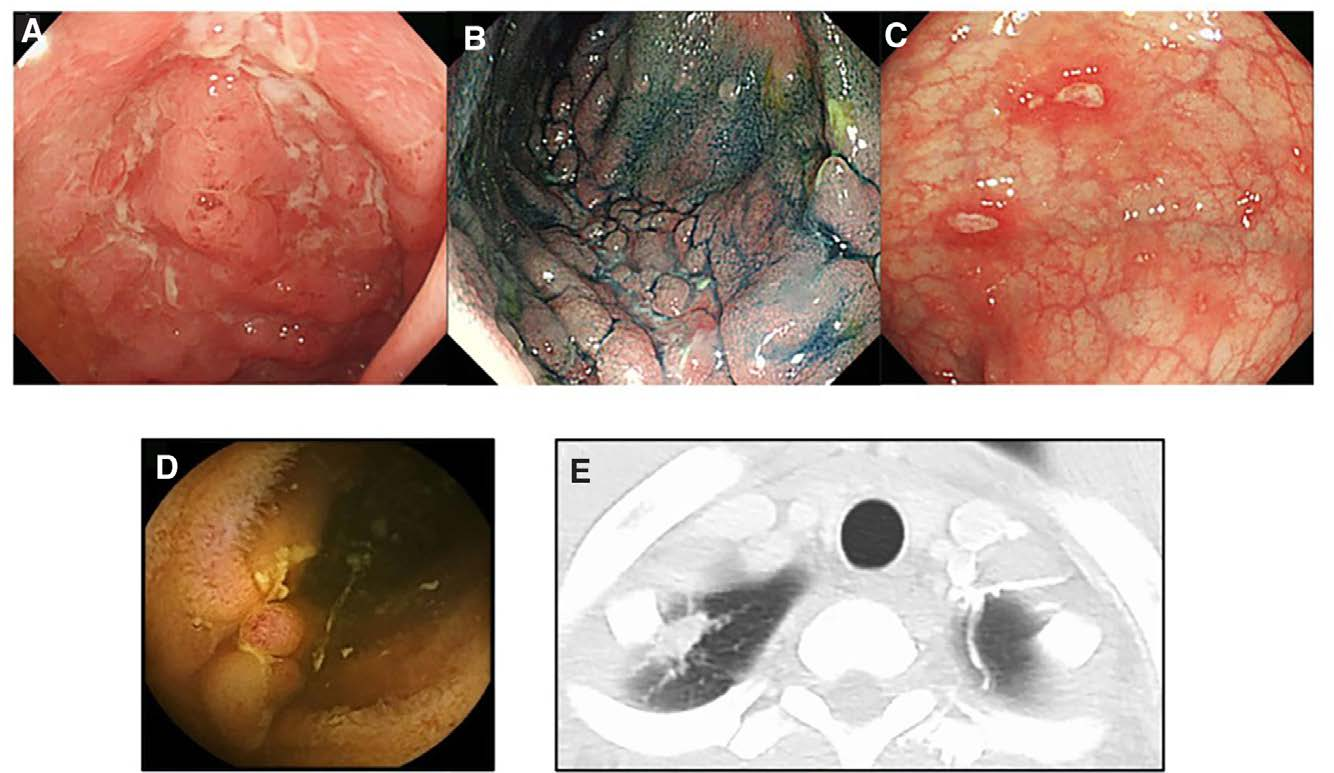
(A–C) Colonoscopic findings: multiple longitudinal ulcers and a cobblestone appearance in the terminal ileum and multiple small aphthae in the colon at the initial diagnosis. (D) Small bowel capsule endoscopic findings: a cobblestone appeared in the ileum at the initial diagnosis. (E) Chest computed tomography findings: a nodular lesion at the apex of the right lung before the administration of anti-TNF-alpha agent.
